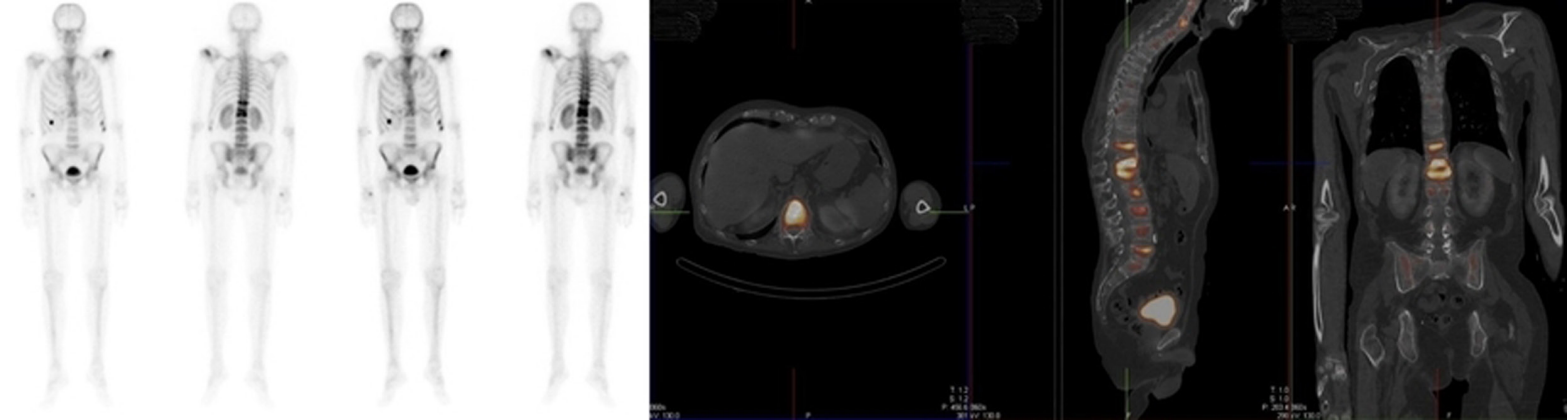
Whole body scintigraphy showing recent vertebral compression fractures at T11, T12, and L1 and semi‐recent factures at the L2, L3, L4, and L5 levels. The scintigraphy also showed rib fractures at the level of the 6th right anterior costal arch and the 7th and 8th left anterior costal arches.
